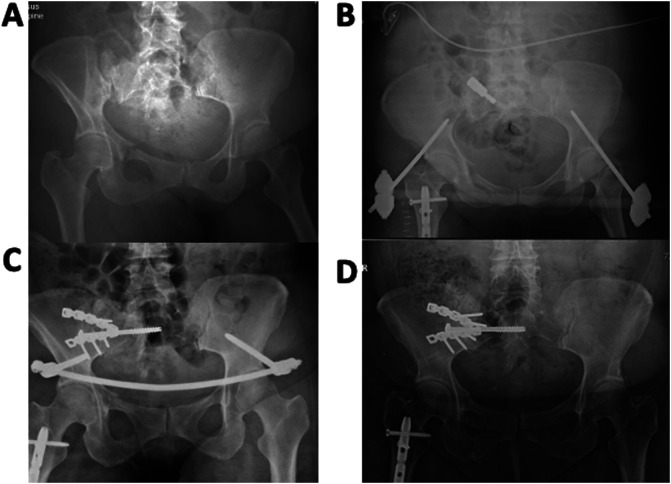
In one case, a supra-acetabular external fixator was used, and the resulting INFIX got infected despite using different holes for the implants. (a) Preop X-ray, (b) with temporary external fixator, (c) after definitive fixation, (d) at last follow-up.
